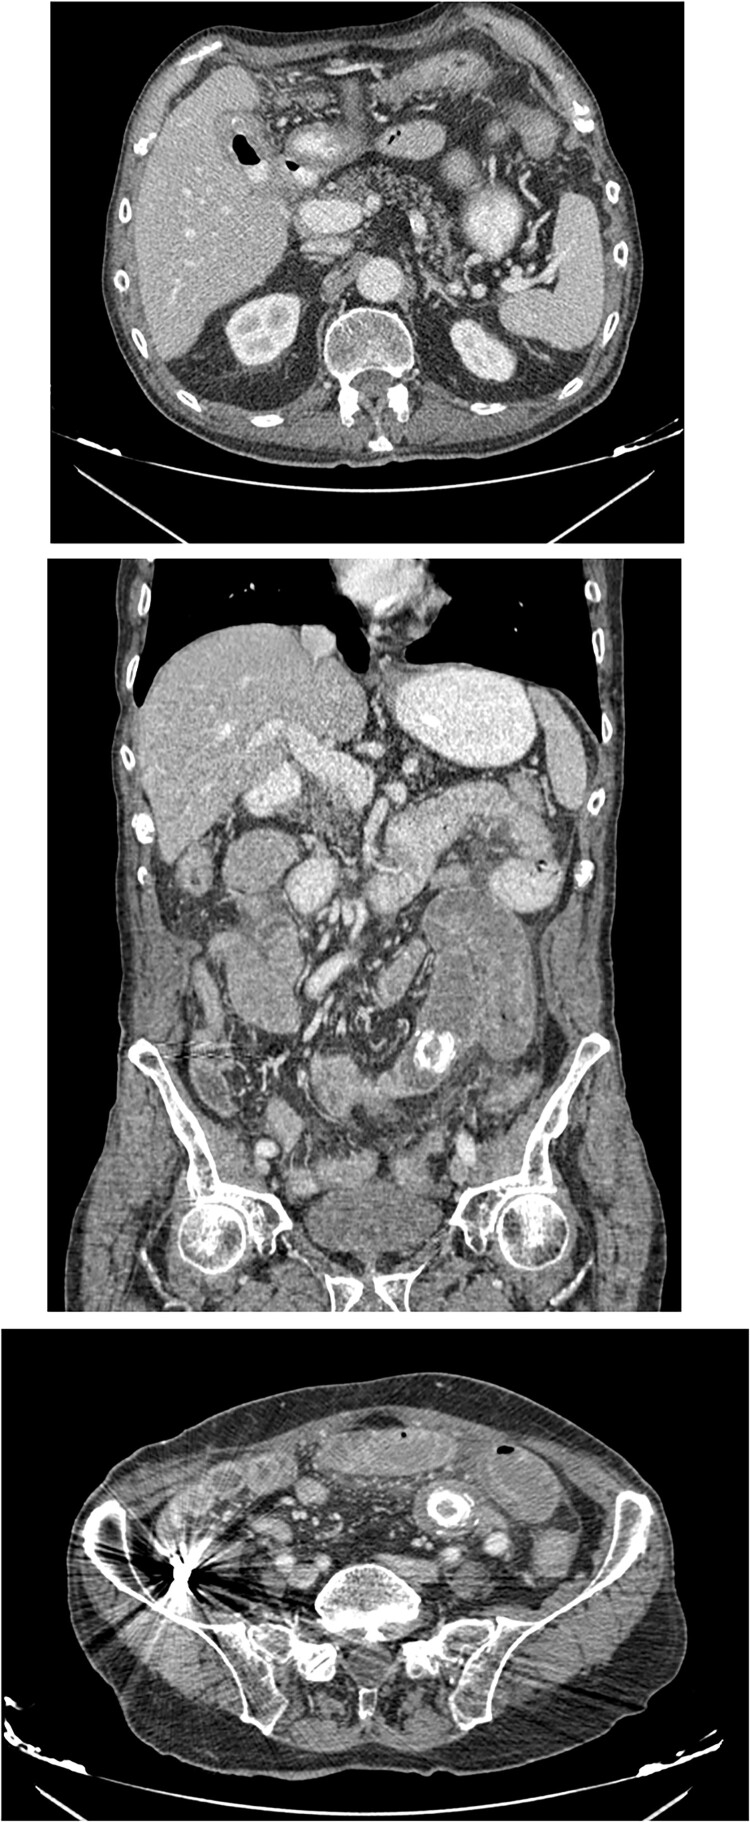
CT images with oral and IV contrast revealed presence of air and oral contrast in the gallbladder and small bowel obstruction caused by a mass in the jejunum–ileum transition attributed to a gallstone.
